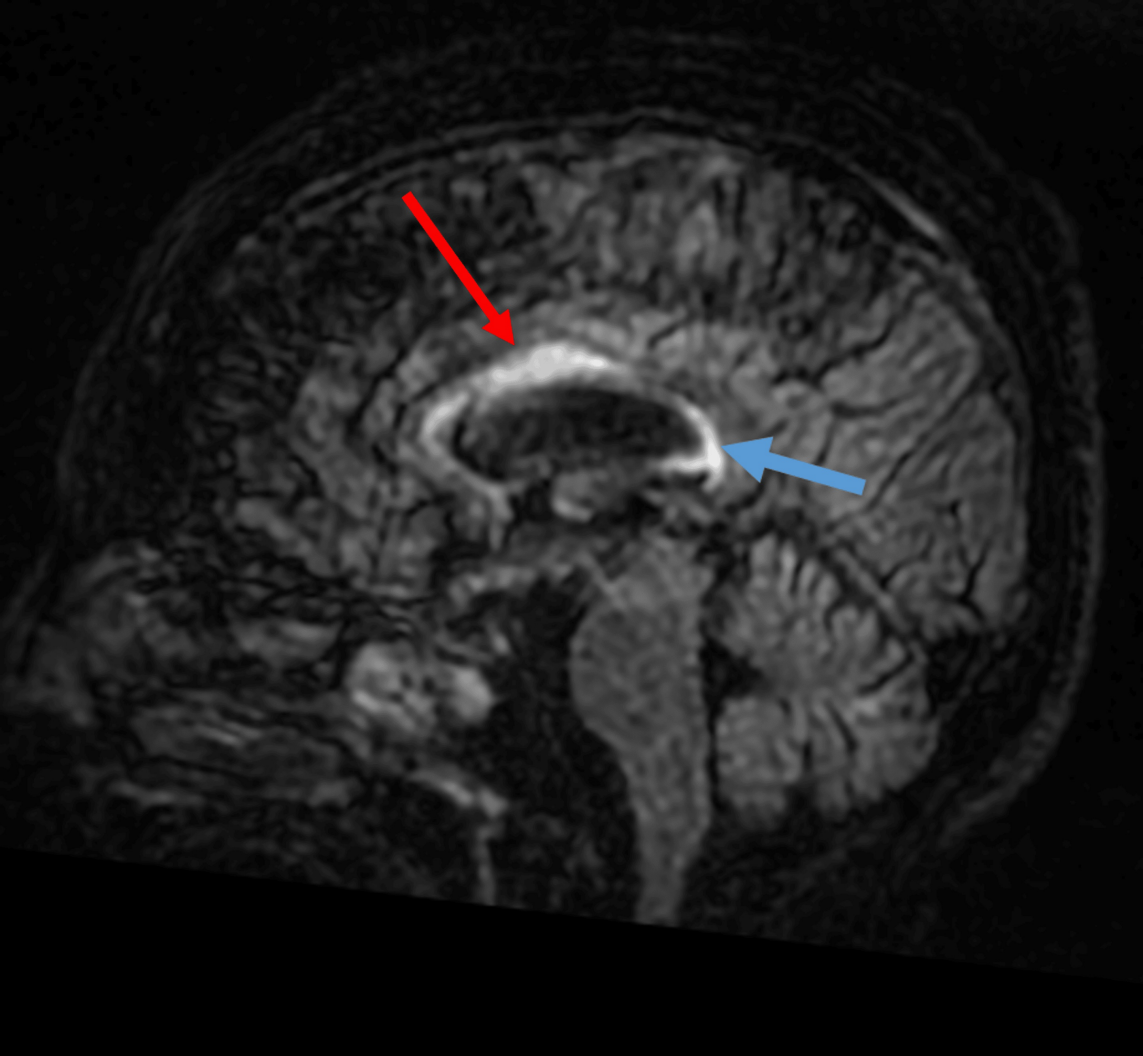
Acute demyelination due to Marchiafava-Bignami disease: MRI T2/FLAIR, sagittal MRI: Magnetic resonance imaging; FLAIR: fluid-attenuated inversion recovery Increased signal intensity affecting the anterior 2/3 of the corpus callosum, with slight associated expansion (red arrow), weaker but increased signal intensity in the splenium of the corpus callosum, but without expansive effect (blue arrow)
